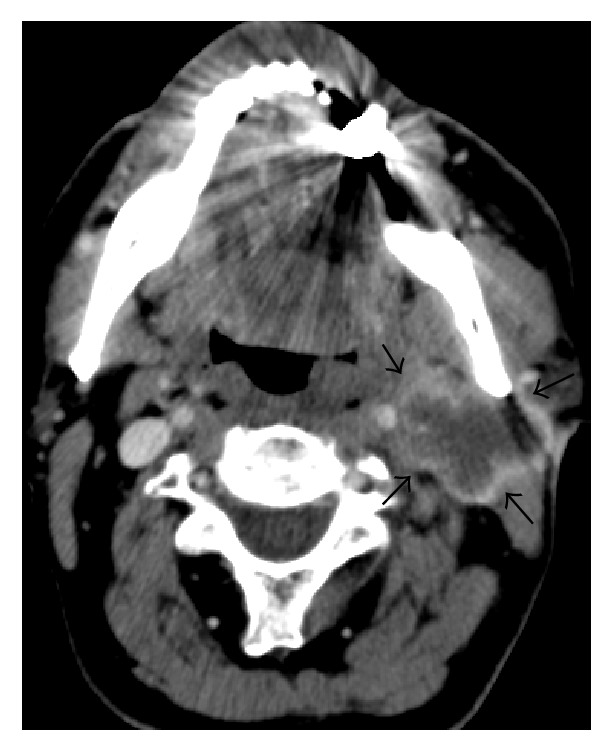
Axial postcontrast CT image of the neck showing left lymphadenopathy with necrotic center and peripheral enhancement.
